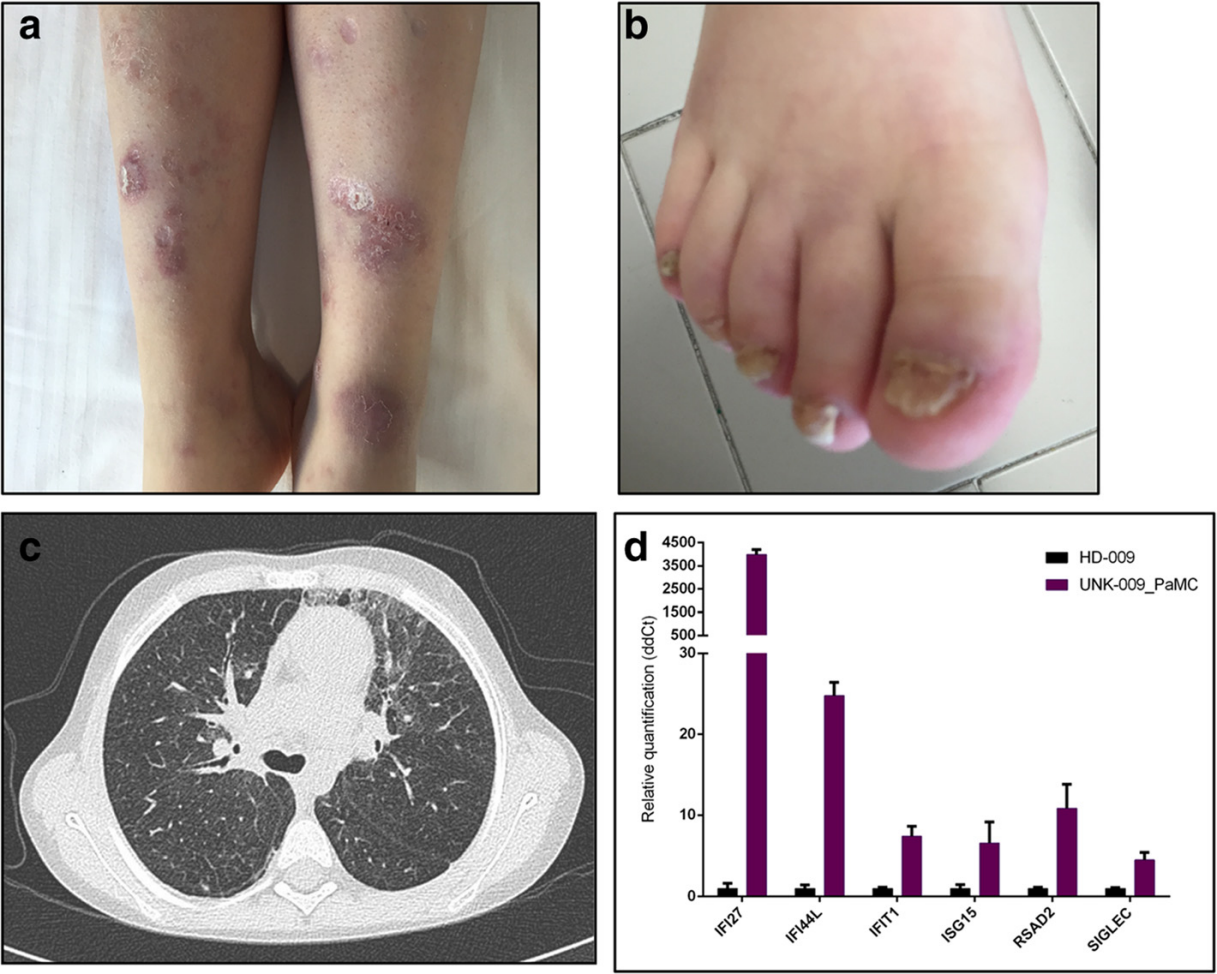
Clinical presentation and blood interferon signature of a SAVI patient. Purpuric plaques with ulcerative evolution (panel a), onychodystrophy (panel b), CT scan revealing focal thickening of the interlobular septa with areas of ground glass opacities (panel c), and peripheral blood type I interferon signature (panel d) (assessed as described [67]) in a patient with SAVI syndrome
